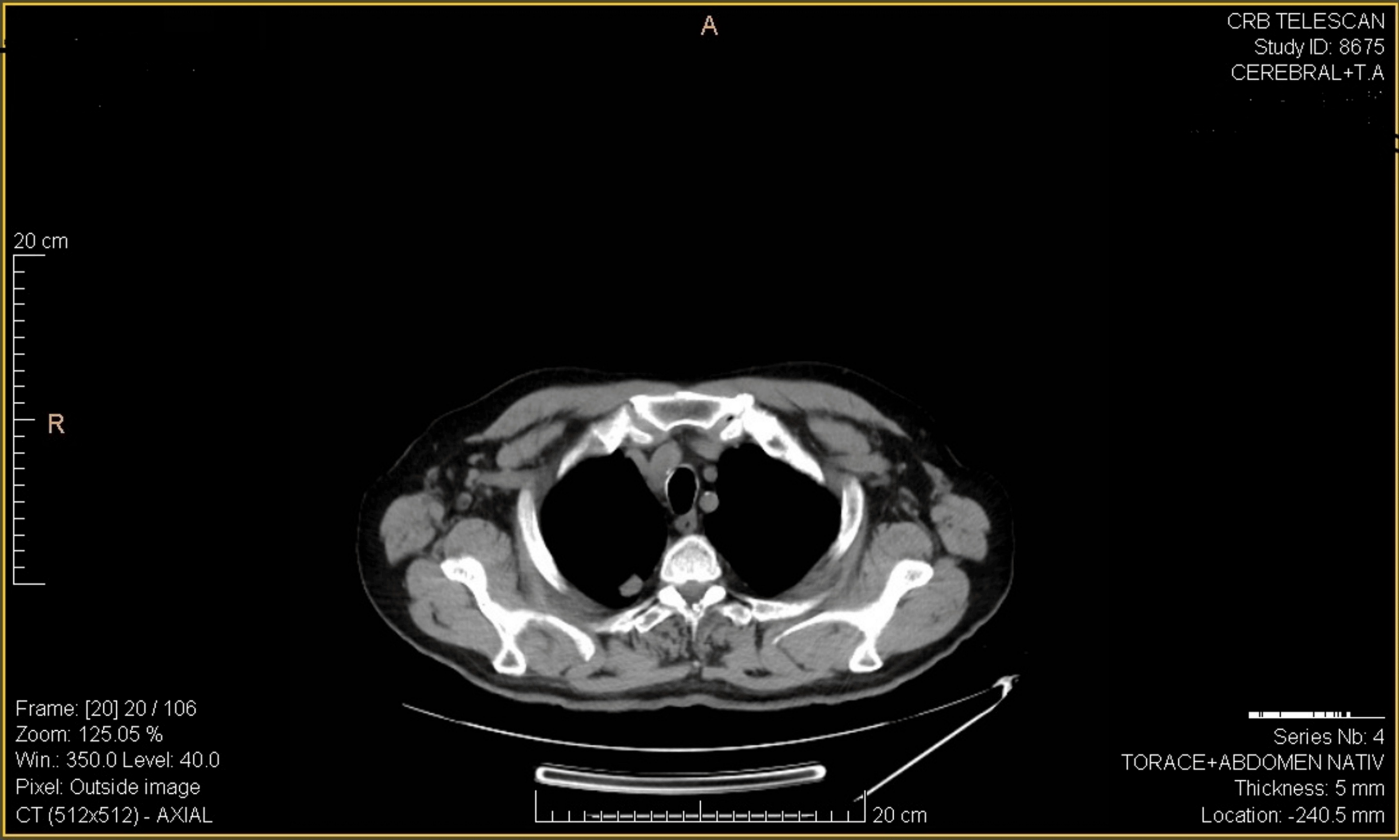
CT scan showing subpleural nodular lesion posterior to the right inferior lobe
